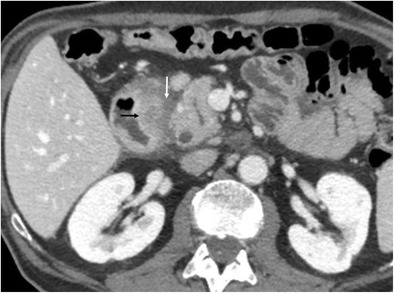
A 48-year-old man with epigastric pain. Contrast-enhanced CT identifies subtle infiltration of soft tissue (white arrow) in the pancreaticoduodenal groove. Duodenal mural thickening secondary to edema is observed on the medial side (black arrow). Note the normal enhancement of the pancreatic head and absence of classic signs of exsudative pancreatitis
